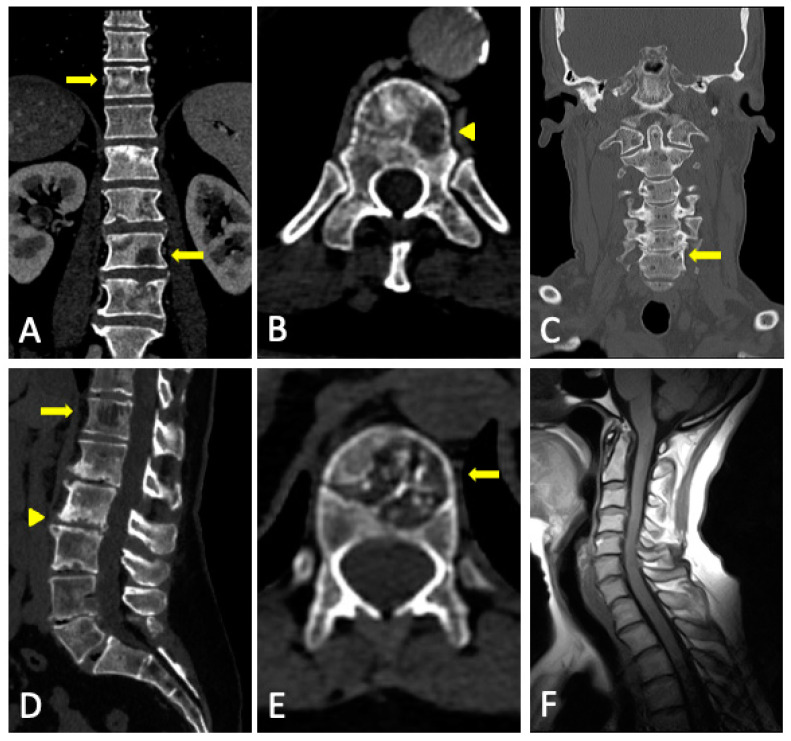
Differential diagnosis in conventional CT. Multiple osseous lesions (arrows in (A)), some of them with mixed pattern and sclerotic rim (arrowhead in (B)) corresponding to lung cancer metastases, incompatible with untreated MM. Angioma in T12 vertebral body (arrows in (D,E)) with intralesional fat and intralesional thick trabeculae conforming to the typical “jail bar pattern” in sagittal reconstruction (D) and “polka dot pattern” in the axial plane (E). Degenerative disc disease in lumbar spine with multiple Schmörl nodules (arrowhead in (D)). We present a case of osteoporosis with multiple millimetric lytic lesions in coronal reconstruction of cervical spine (C), some of them with sclerotic rim (arrow in (C)) with a normal signal of the bone marrow in T1WI sequence (F) and no findings of hematologic cell dyscrasia in laboratory tests.
